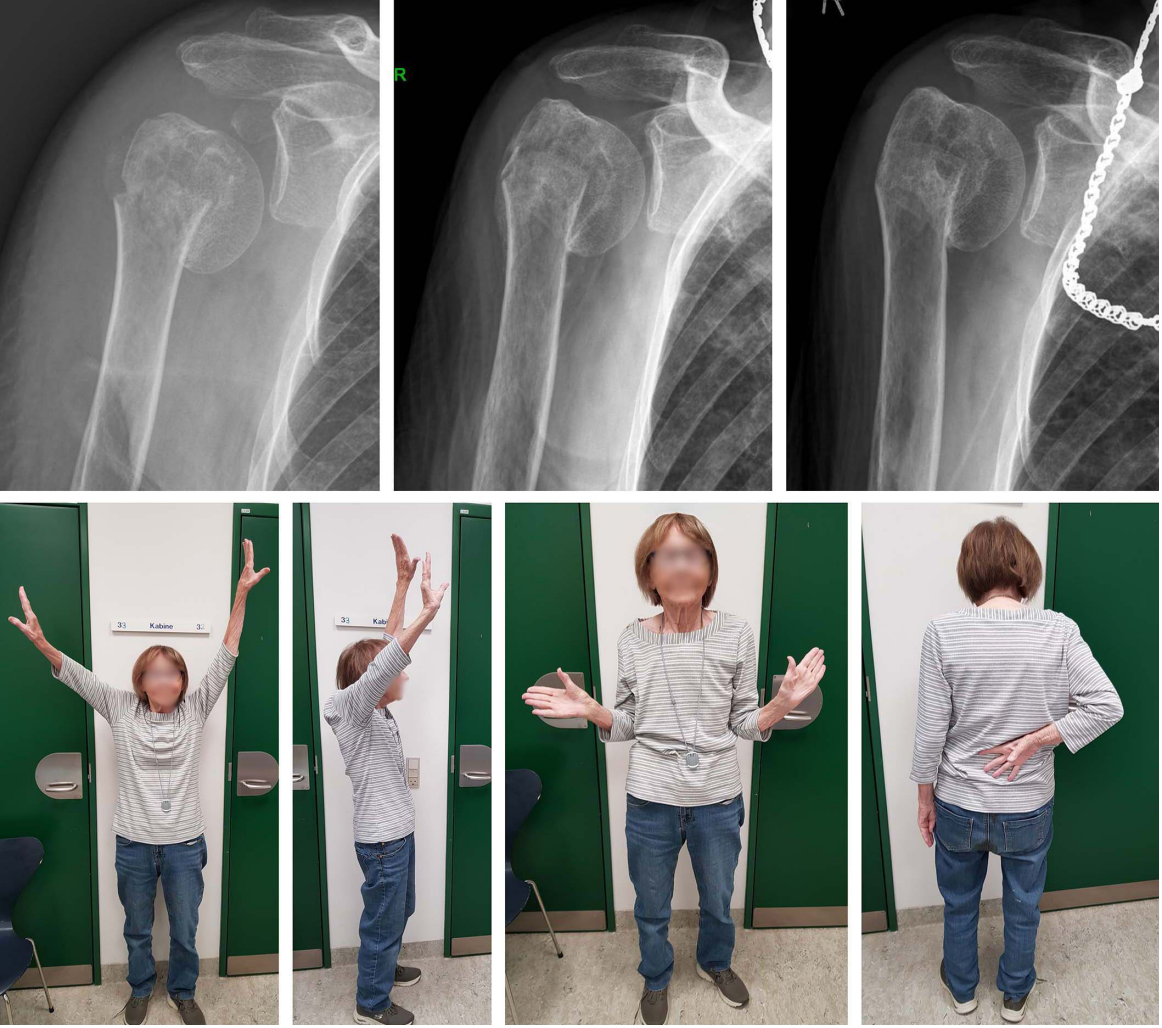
An 89-year-old nursing home resident with a varus displaced 2-part surgical neck fracture. The fracture healed in varus malunion, but the patient obtained pain-free shoulder function. Radiographs at admission, 6 weeks, and 24 weeks. Clinical photos at 24 weeks.
